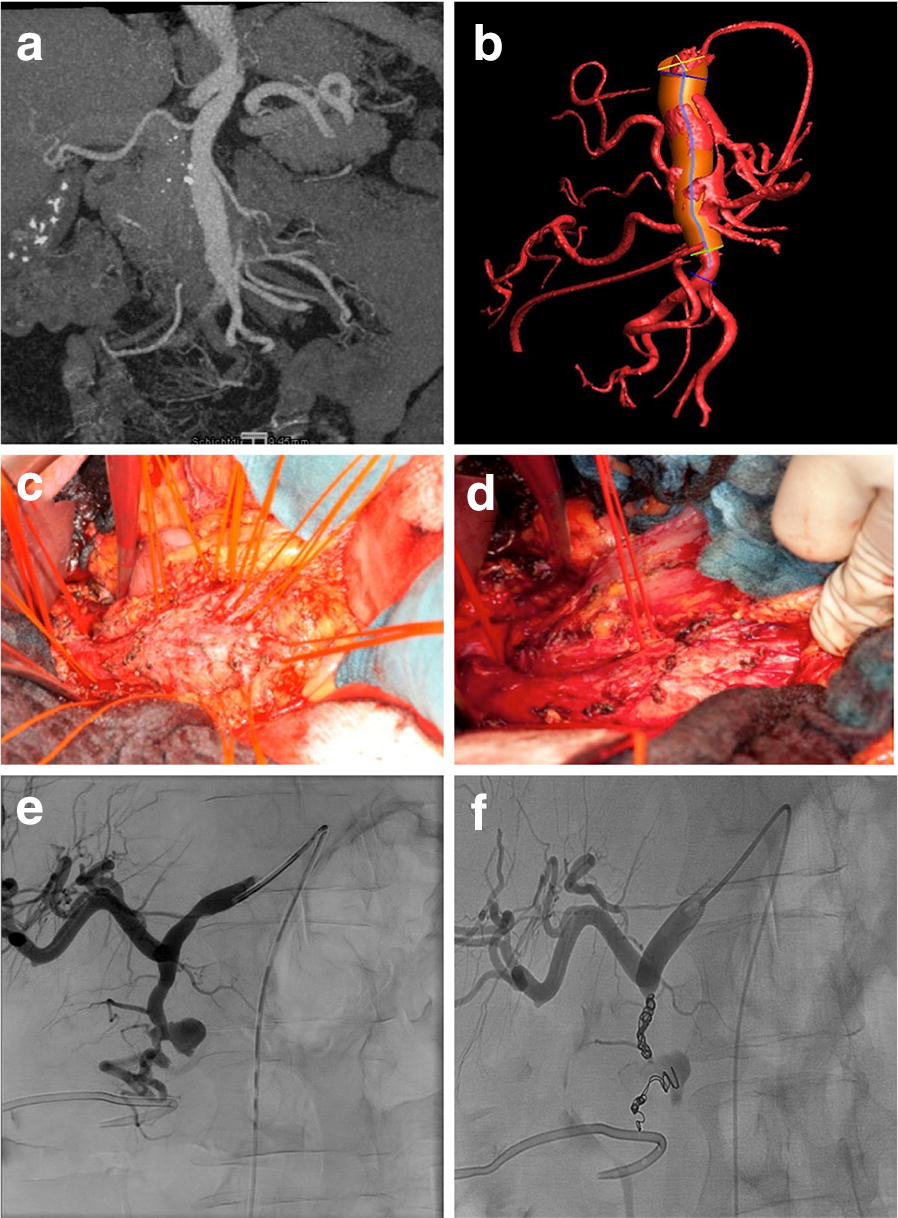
CT scan of a 62-year-old patient with a big asymptomatic SMA aneurysm (a). Digital reconstruction of the CT scan in a (b). Intraoperative images of the same patient before (c) and (d) after isolation of 14 collateral branches. Angiography of a 37-year-old patient with an aneurysm of the gastroduodenal artery after perforation (e). Same patient in e after a successful coiling procedure (f)
